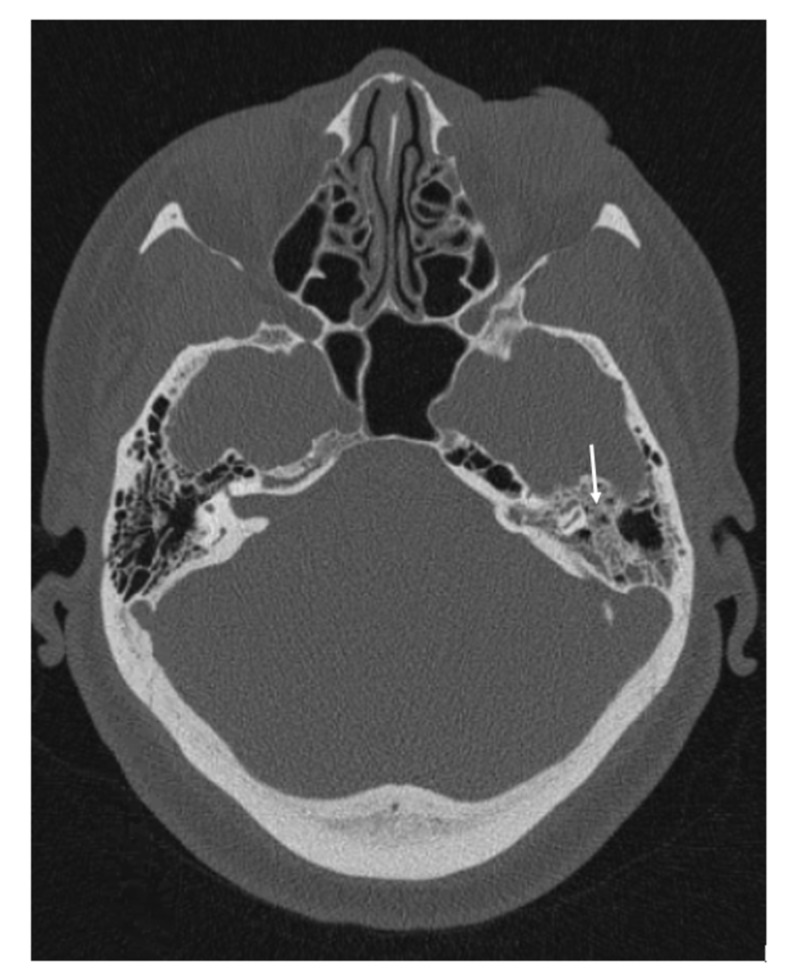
Computed tomography scan of the internal auditory canal (IAC) without intravenous (IV) contrast demonstrating fluid in the middle ear and multiple left mastoid sinus air cells (white arrow) consistent with otomastoiditis.
